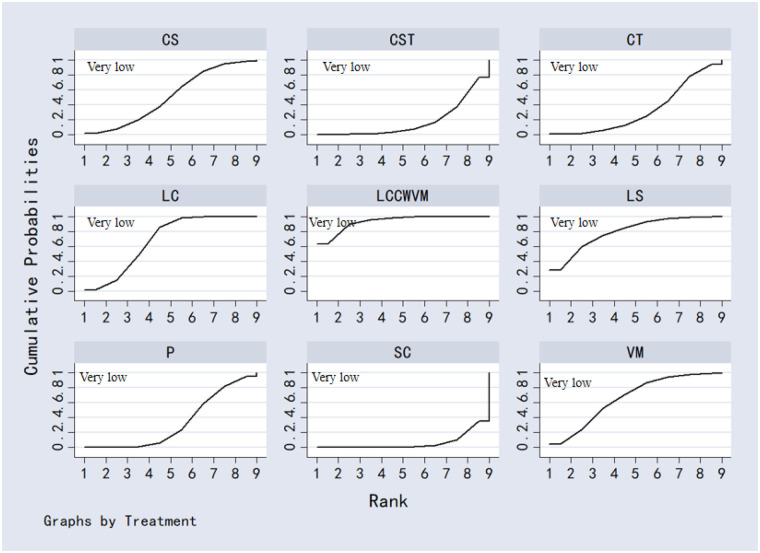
The SUCRA of the pain score. SUCRA, surface under the cumulative ranking; LC, lidocaine cream; S, lidocaine spray; CS, cold spray; CT, cryotherapy; CST, cutaneous stimulation therapy; LCCWVM, lidocaine cream combing with valsalva maneuver; P, placebo; SC, standard care; VM, valsalva maneuver.
